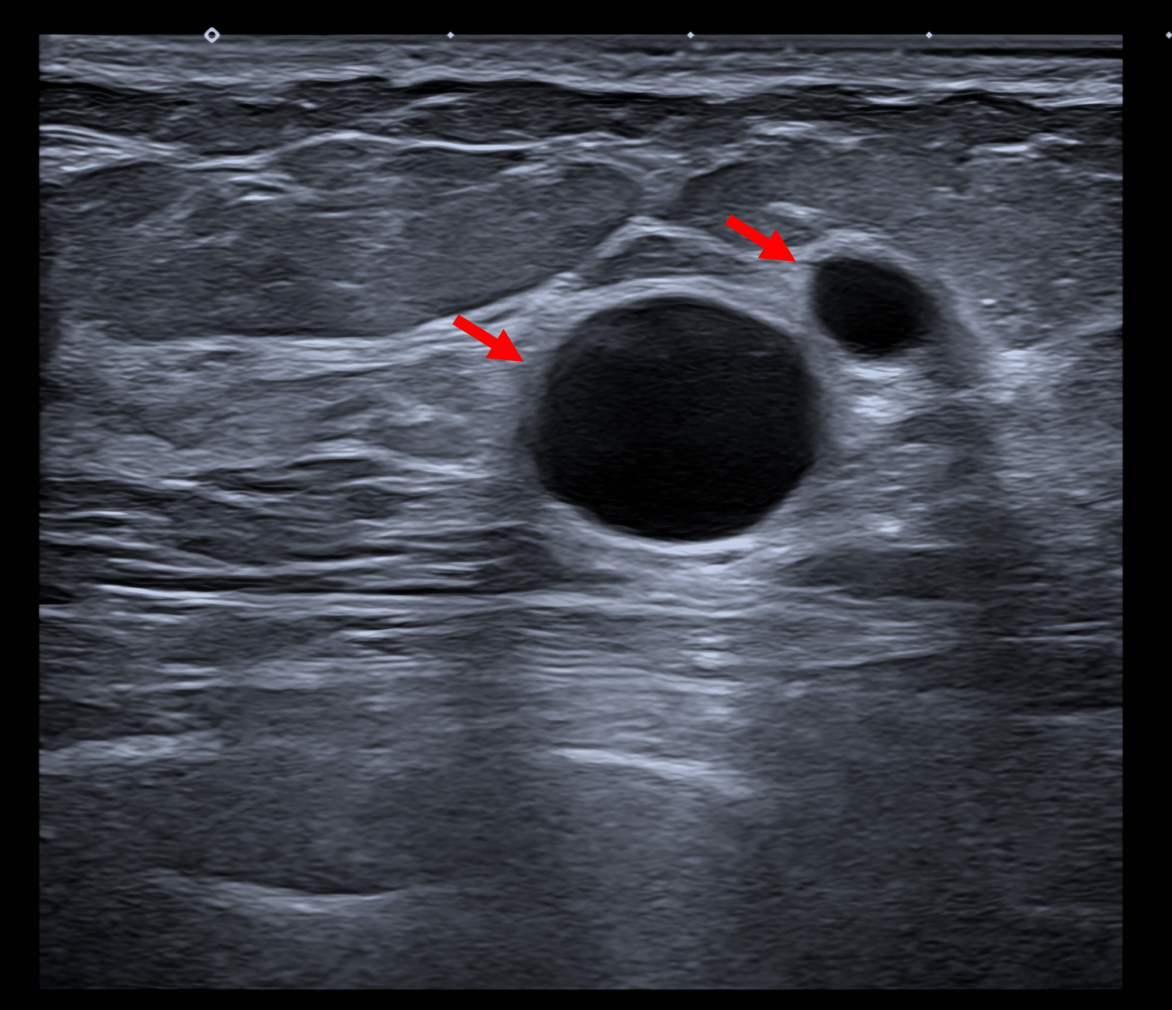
Left breast ultrasound Red arrows indicate multiple benign cysts
